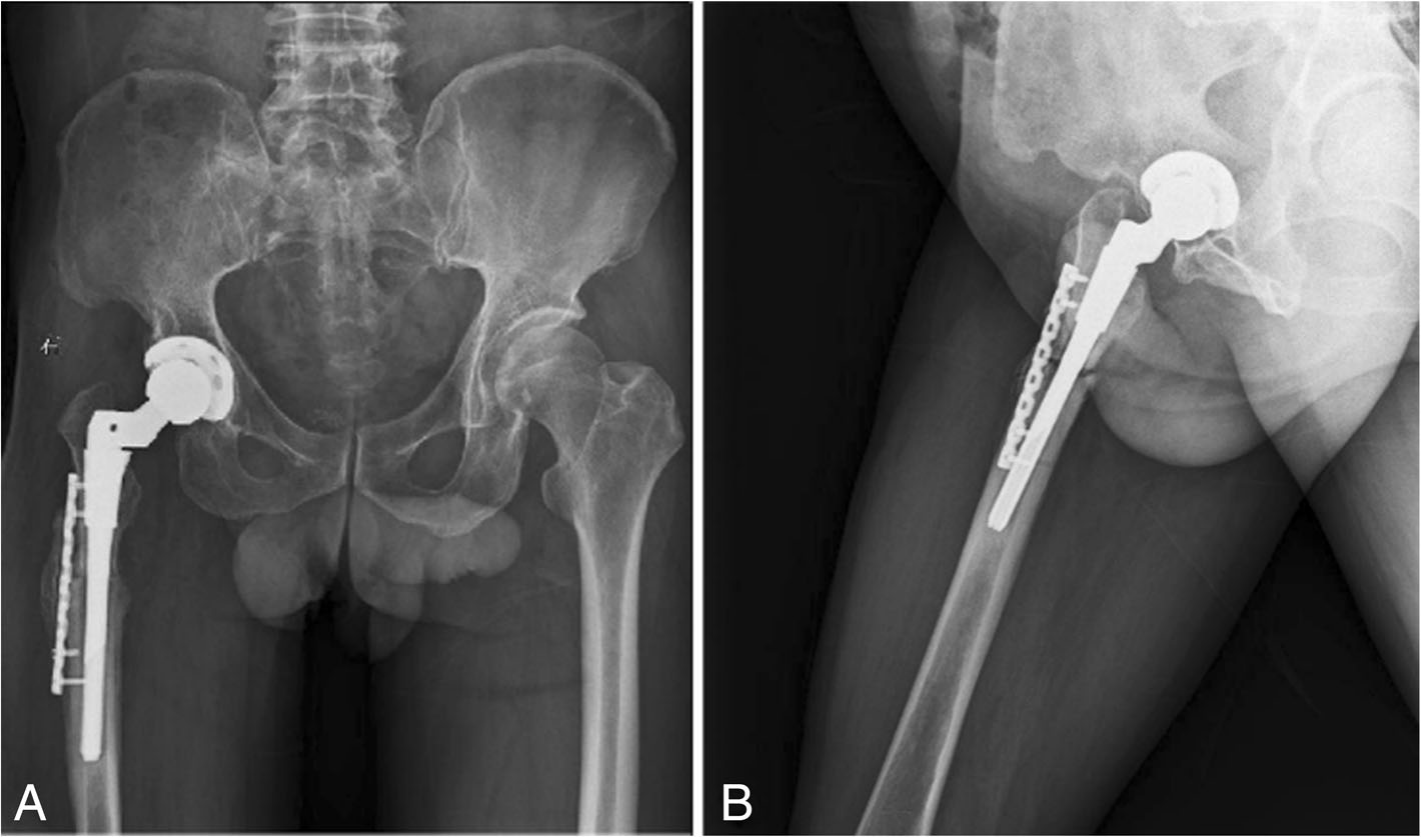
Radiographs of the right hip at 8 months after internal fixation was applied. (a) anteroposterior view of the right hip, (b) lateral view of the right hip
